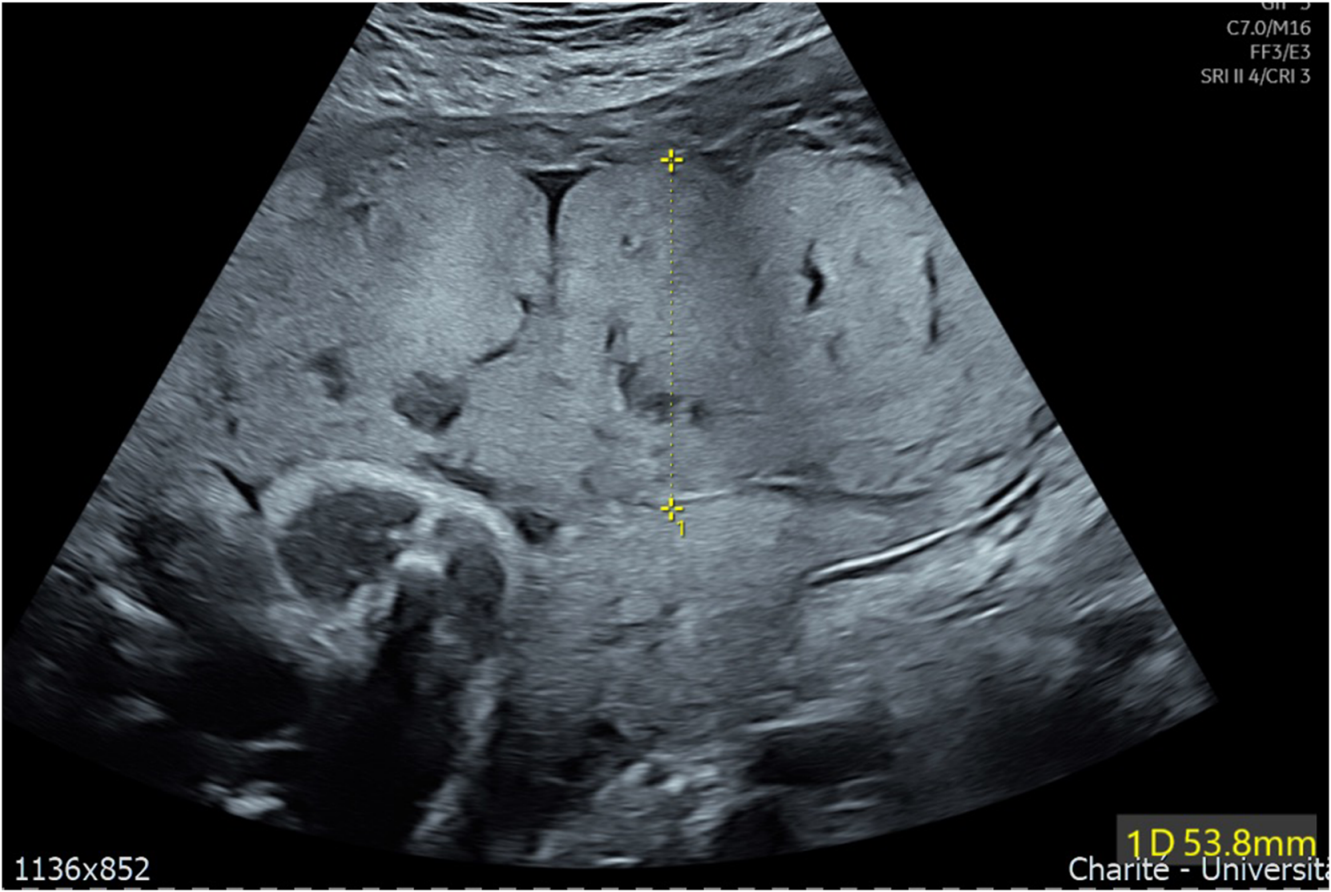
B-mode ultrasound of the placenta at 30 + 6 weeks’gestation showing a thickened placenta of more than 5 cm and multiple lacunae.
